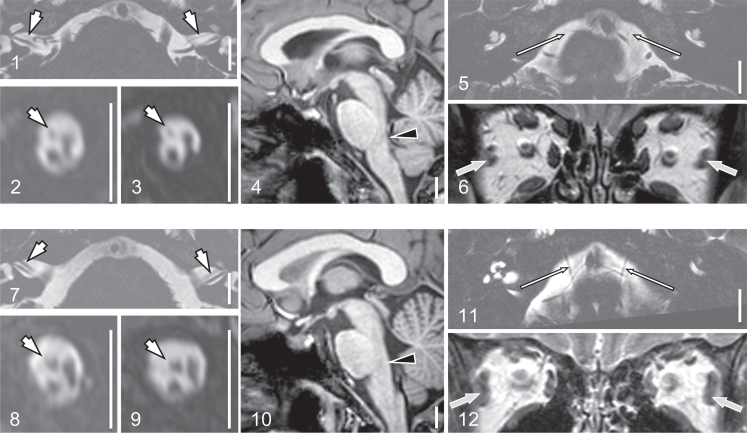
Brain magnetic resonance imaging findings in MBS. Features of MBS on brain MRI from a 33-year-old female proband with MBS and clubfoot (F58-001, panels 1-6) compared with a 24-year-old individual with hemifacial myohyperplasia in the absence of MBS and intracranial abnormalities (panels 7-12). CN7 (short white arrows in 1-3 and 7-9) is hypoplastic in MBS (1-3) but is well seen in the control (7-9). The pontine tegmentum (black arrowhead in 4) is hypoplastic in F58-001, resulting in a concavity in the dorsal brainstem. CN6 cannot be identified in the MBS proband (long white arrows in 5) but is well seen traversing the prepontine cistern in the control (long white arrows in 11). The lateral rectus muscles are relatively small (gray arrows in 6) in MBS compared with control (gray arrows in 12). Imaging sequences: T2 weighted volume isotropic turbo spin echo images reformatted axial through the internal auditory canals (1,7) and oblique axial through the prepontine cisterns (5,11); sagittal reformats of 0.5 mm isotropic balanced fast field echo through the right (2,8) and left (3,9) IACs at sites of arrows in panels 1 and 7; midline sagittal image from 1mm isotropic 3D (4,10); coronal fast spin echo T2 through the orbits (6,12). Scale bars, 1 cm (white bars in 1-5 and 7-11). See also Supplemental Figure 3. MBS, Moebius Syndrome.
